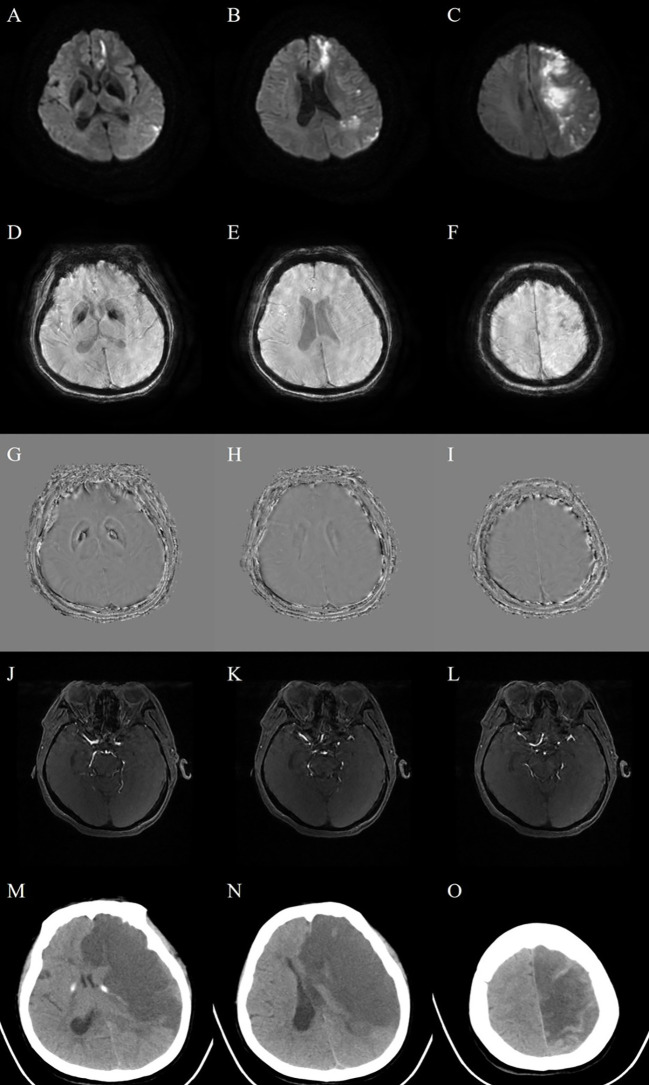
Neuroimaging findings at admission and during clinical deterioration. (A–C) Admission diffusion-weighted imaging (DWI): Hyperintense signals are evident in the left frontoparietal cortex and deep gray nuclei, consistent with acute ischemic infarcts involving multiple vascular territories. (D–I) Susceptibility-weighted imaging (SWI): Focal susceptibility blooming artifacts within the infarcted regions indicate hemorrhagic transformation with microhemorrhages. (J–L) Axial MRA source images demonstrated ≥50% luminal narrowing in the M1 segment of the left middle cerebral artery. (M–O) Emergency cranial computed tomography (CT) on day 16: Worsening hypoattenuation in the left hemisphere reflects progressive infarction, accompanied by effacement of the left lateral ventricle and subfalcine herniation.
